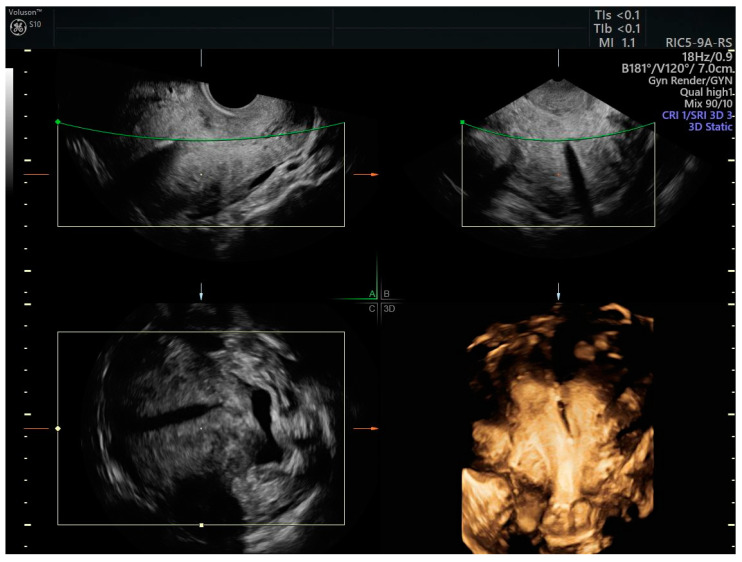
3D scan of the uterus of a patient with endometrial cancer treated with LNG-IUD. The correct positioning of the intrauterine device is monitored by ultrasound, which also allows the identification of any changes in the endometrial pattern during treatment.
